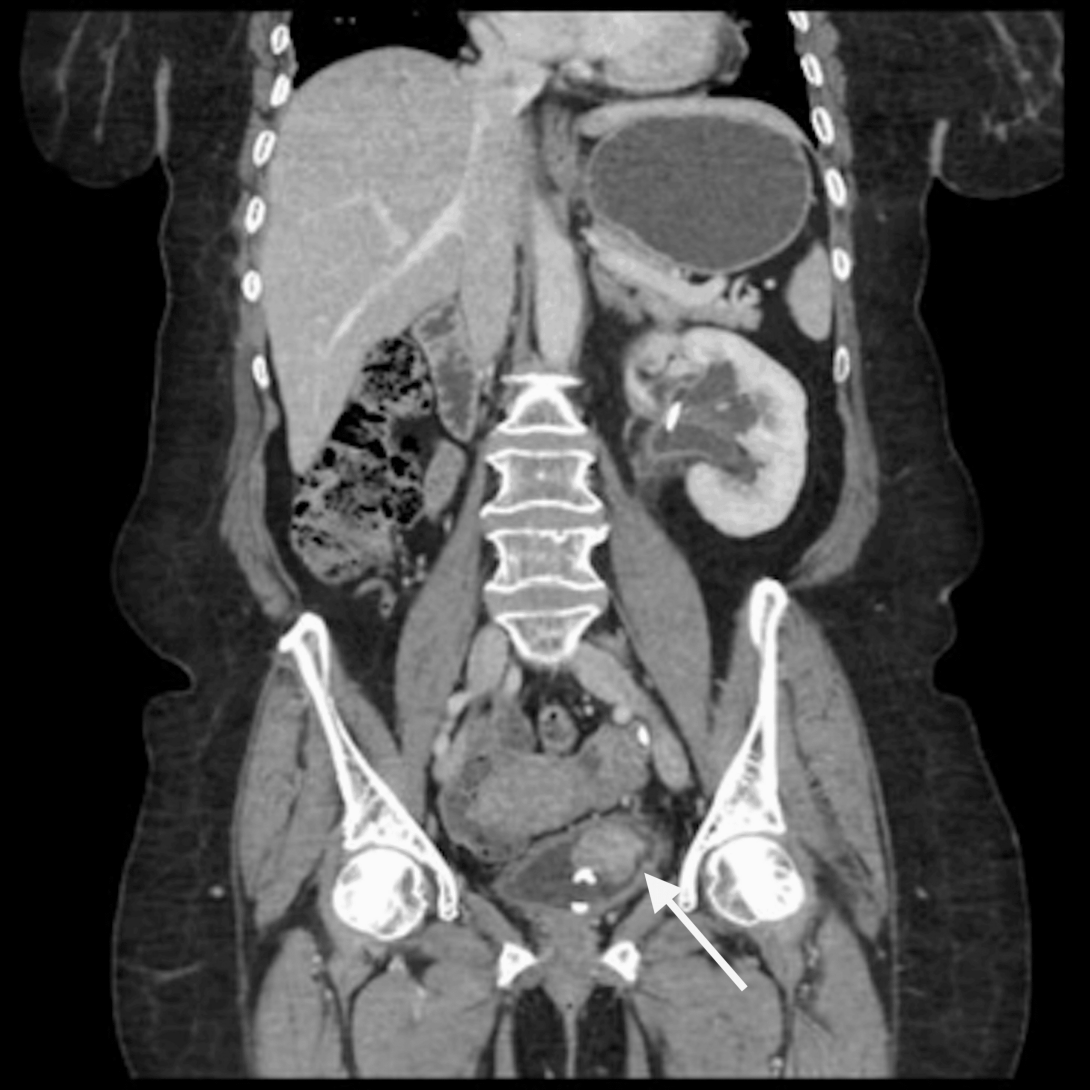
CT urography shows an enhancing mass arising near the left ureteric orifice with a DJ stent in situ.
